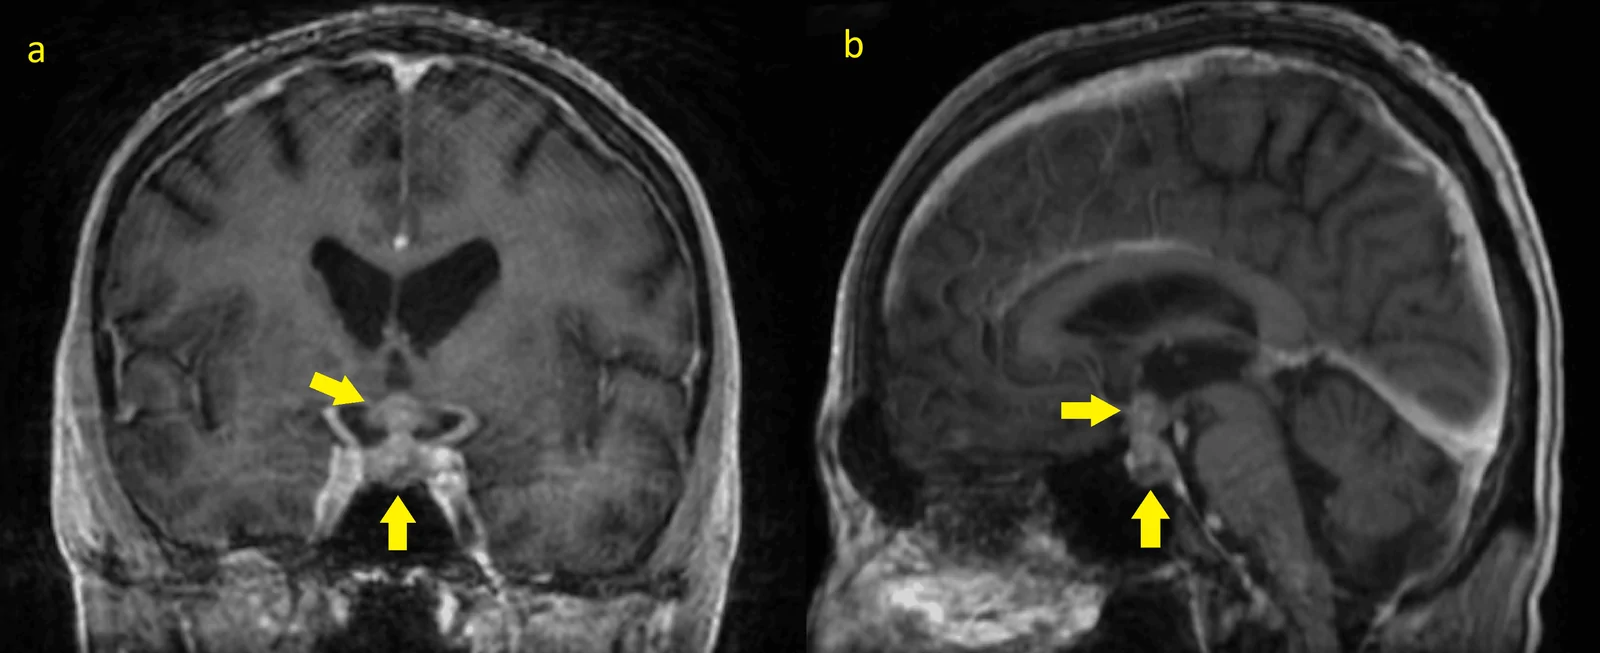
MRI of the brain with contrast demonstrating the bilobed sellar-suprasellar mass The yellow arrows point to each half of the bilobed appearing structure as seen in both (a) coronal and (b) sagittal views.
